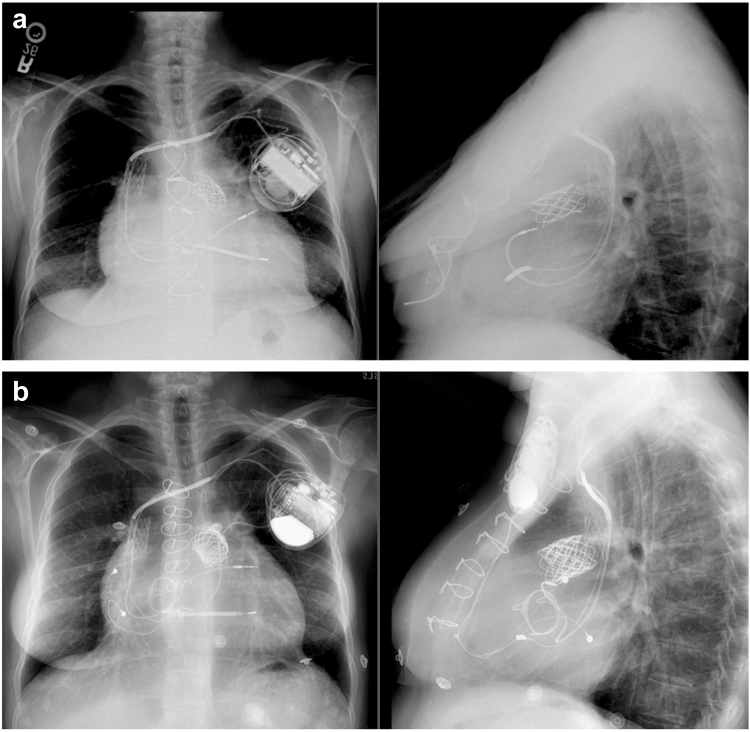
(a) Posteroanterior/lateral chest X-ray from a patient with tetrology of Fallot with atrial and ventricular arrhythmias who had a hybrid endocardial and epicardial defibrillator implanted. There are also superior vena cava, left pulmonary artery, and right ventricular outflow tract stents with a transcaether pulmonary valve replacement present. (b) Posteroanterior/lateral chest X-ray after a bioprosthetic tricuspid valve replacement (27 mm Magna Ease) demonstrating a defibrillator lead jailed by both the tricuspid valve replacement and the superior vena cava stent.
